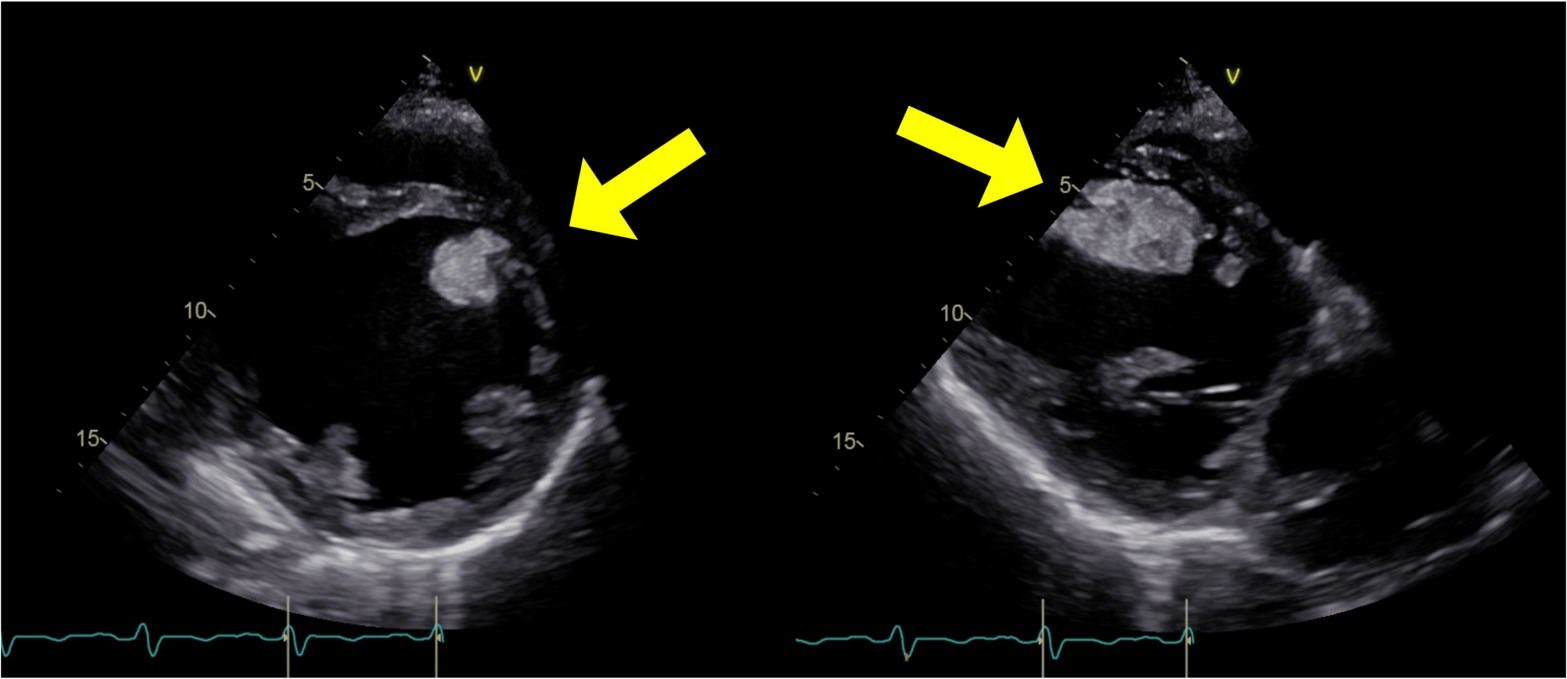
Transthoracic echocardiography The left panel shows a short-axis view of the left ventricle, and the right panel displays a long-axis view. A large, mobile thrombus is clearly visualized within the left ventricular cavity
